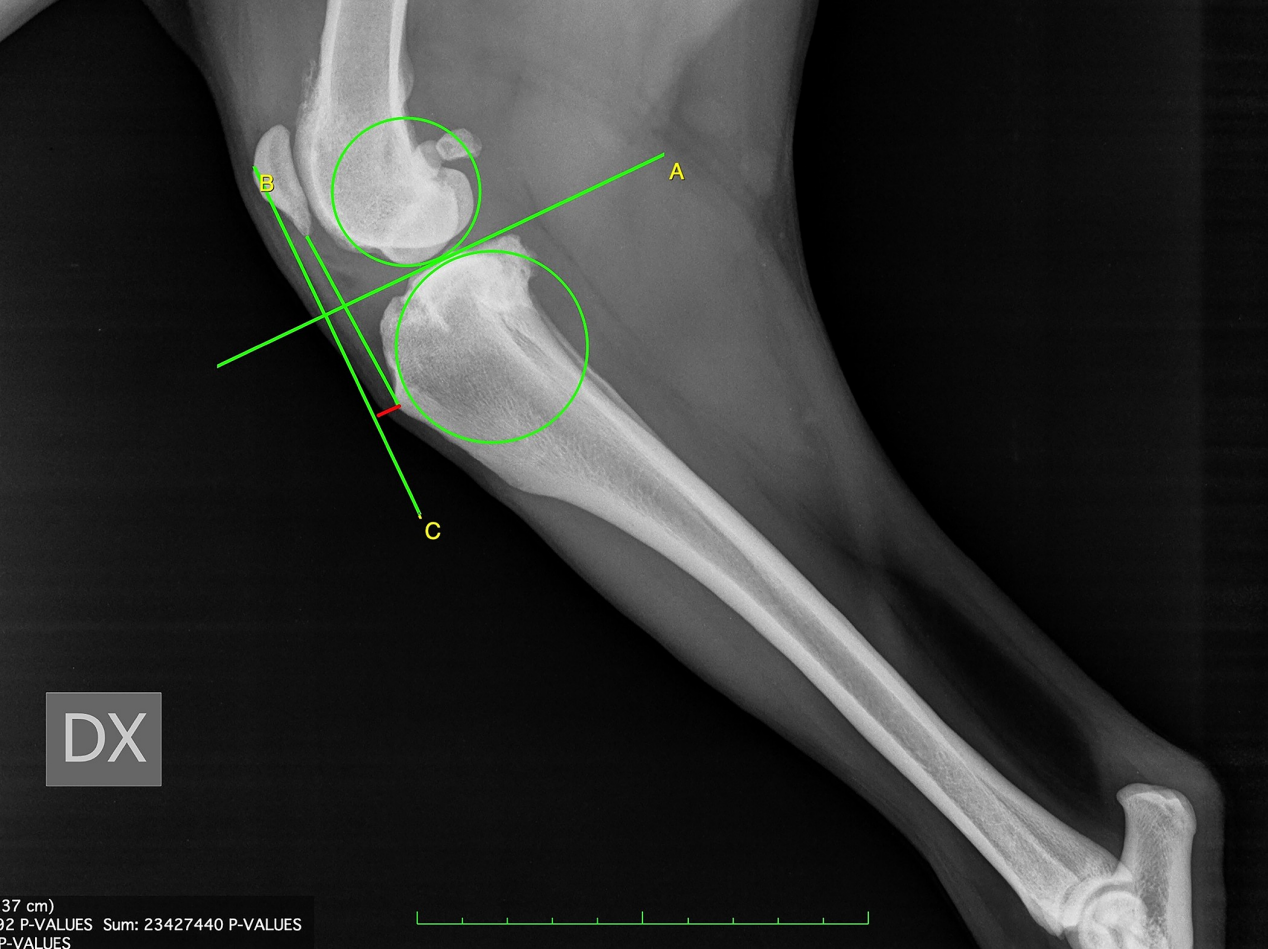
Common tangent (CT) method for the measurement of tibial tuberosity advancement.
